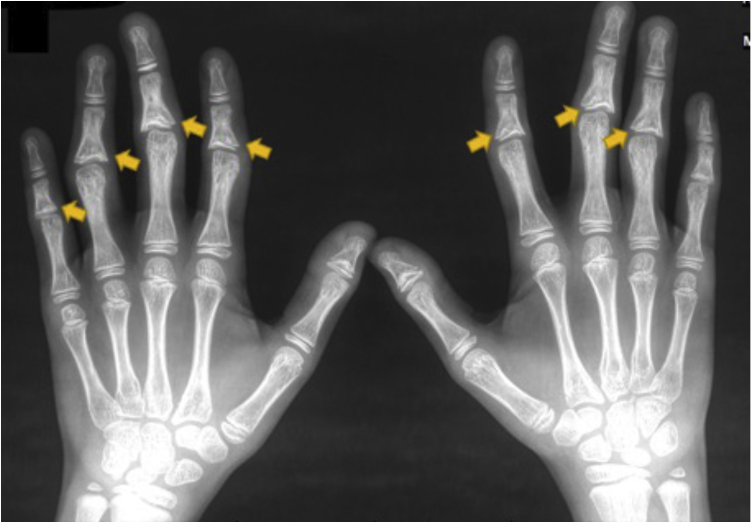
Hand x-ray showing coned epiphyses in the middle phalanges (yellow arrows).
